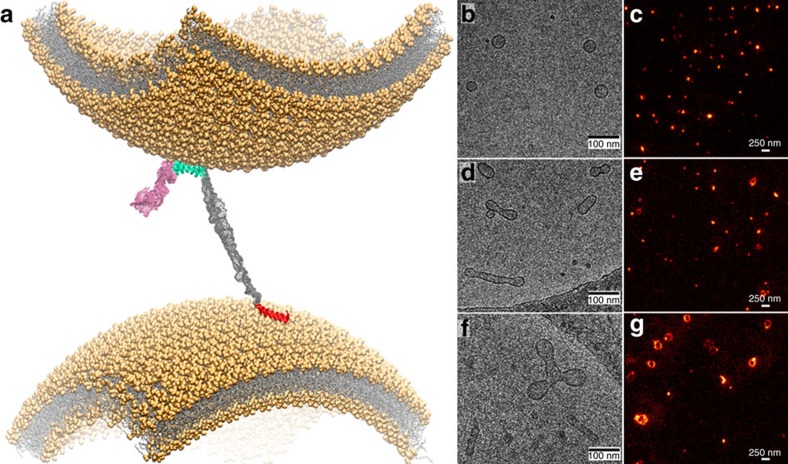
Vesicle assembly induced by αS. (a) Molecular details of the double-anchor mechanism described in this work. SUVs of 50 nm in diameter were modelled to mimic as closely as possible the experimental conditions in this study (see Methods section). αS was modelled with the N-terminal anchor in an amphipathic α-helical conformation (red) and bound to the lower vesicle. The region 65–97 (cyan) of αS was modelled in an amphipathic α-helical conformation bound to the upper vesicle. The C-terminal fragment (residues 98–140) and the linker region 26–59 are shown in pink and grey colours, respectively. With this topology the modelling reveals that a single αS molecule could simultaneously bind two vesicles that are up to 150 Å apart. (b,c) Cryo-EM (b) and STED (c) images acquired on SUVs at a concentration of 0.5 mg ml^−1^. (d,e) Cryo-EM (d) and STED (e) images measured on SUVs following a 12 h incubation with 200 μM αS_WT_. (f,g) Cryo-EM (f) and STED (g) images acquired on SUVs following a 12 h incubation with 200 μM αS_Sw_.
